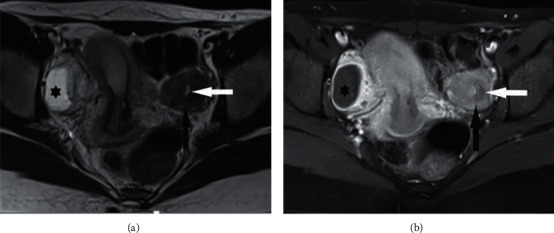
(a) Axial T2-weighted image of the pelvis shows a well-defined left-sided adnexal mass with signal intensity similar to the uterus (black arrow) with a central hypersignal endometrium (white arrow). (b) Axial contrast-enhanced fat-saturated T1-weighted image of the pelvis also demonstrates an enhancement similar to the uterus (black arrow). A right-sided simple ovarian cyst is also observed (asterisk). No pelvic lymphadenopathy is evident on magnetic resonance imaging.
